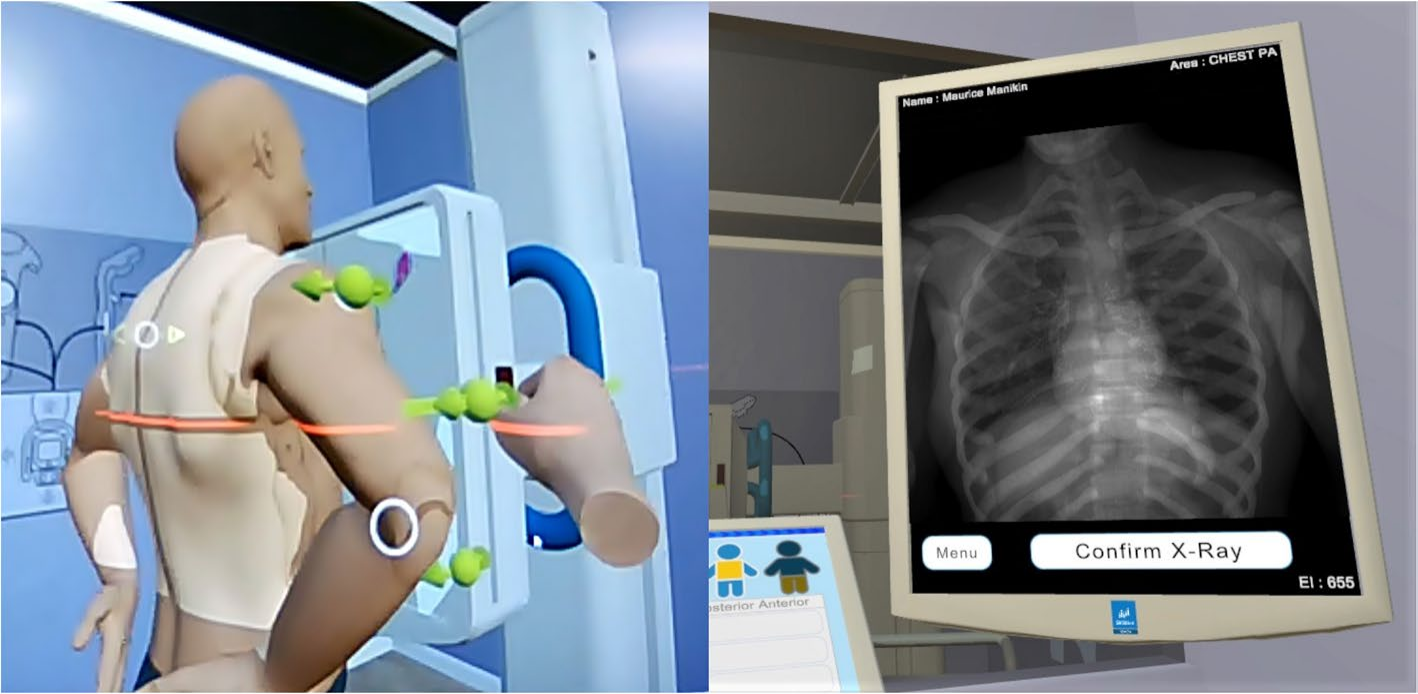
Captured image of patient positioning in VR X-Ray. Users can operate the X-ray equipment and the examinee in a highly immersive VR space. Users can also perform X-ray imaging in the VR space and train while checking the captured X-ray images
